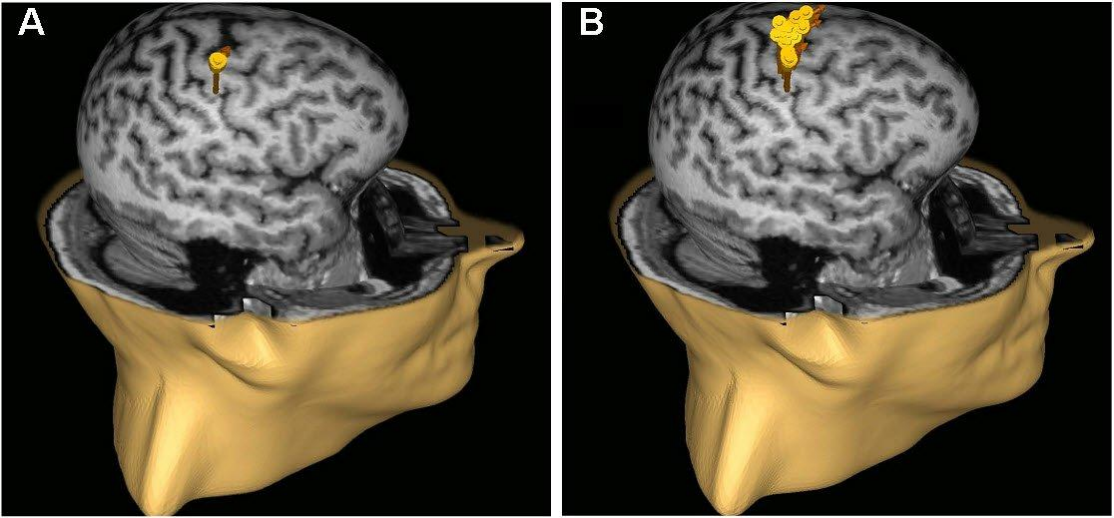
MRI-guided Neuronavigation Allows rTMS to Target the Same Cortex More Precisely and Reproducibly. Panels A and B display a repetitive application of 20 stimuli with (A) and without (B) neuronavigation to the motor cortex area. Note the accuracy of the neuronavigated system (A), compared to the lessprecise application achieved without MRI navigation.
